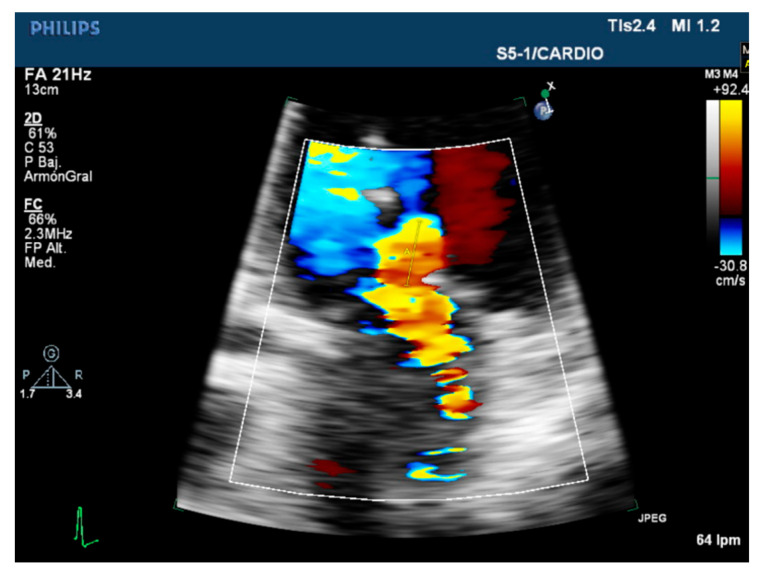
Transthoracic echocardiography. PISA measurement in severe mitral regurgitation.
